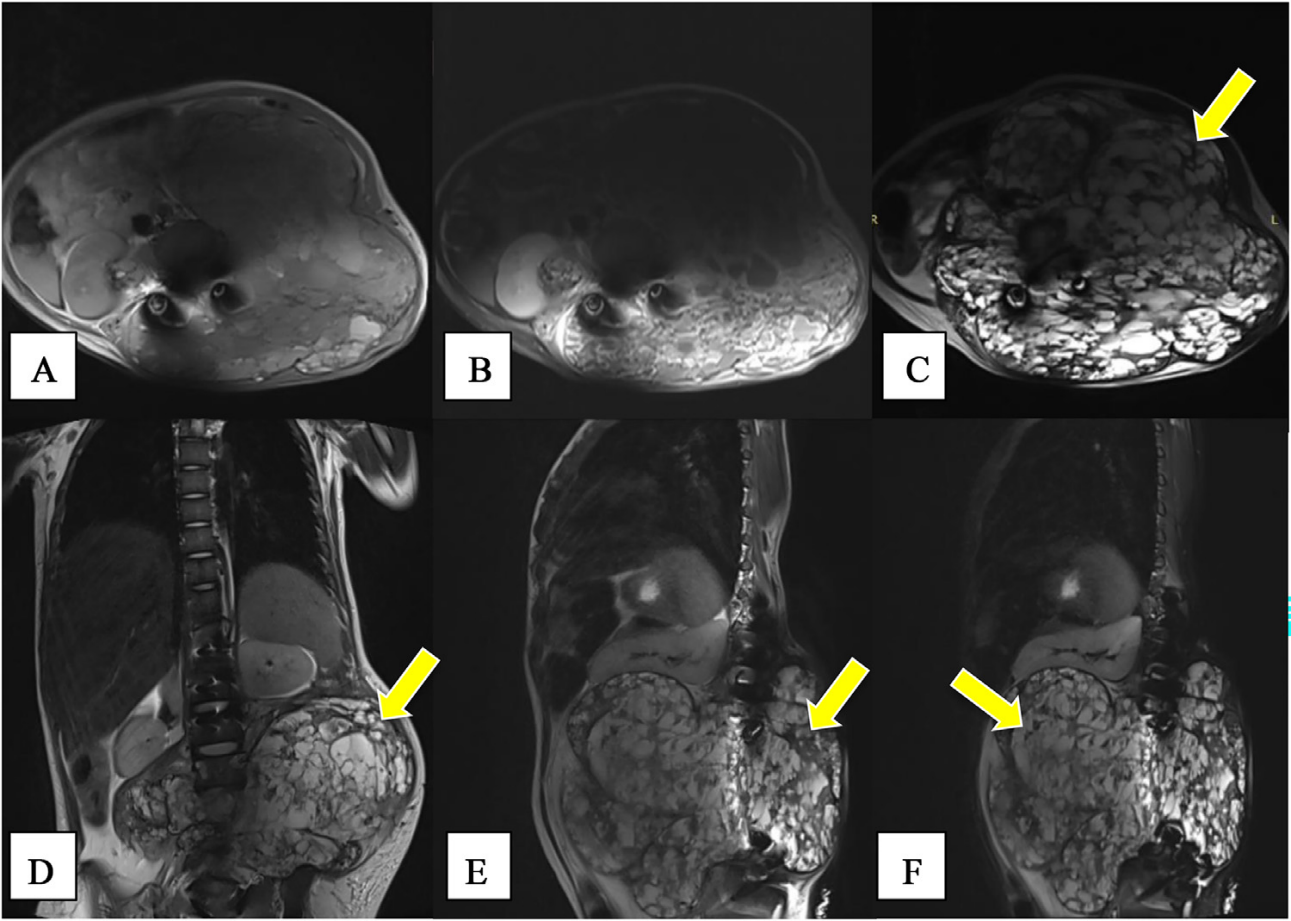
Recurrence after first surgical Magnetic Resonance Imaging (MRI). MRI the lumbosacral region, including T1WI axial noncontrast (A), T1WI axial contrast (B), T2WI axial (C), T2WI coronal (D), T2WI sagittal (E), and T2 Dixon W sagittal (F) showed a sharp transition zone without periosteal reaction formed a “soap bubble appearance” (yellow arrow) surrounding the lumbar paravertebral area.
